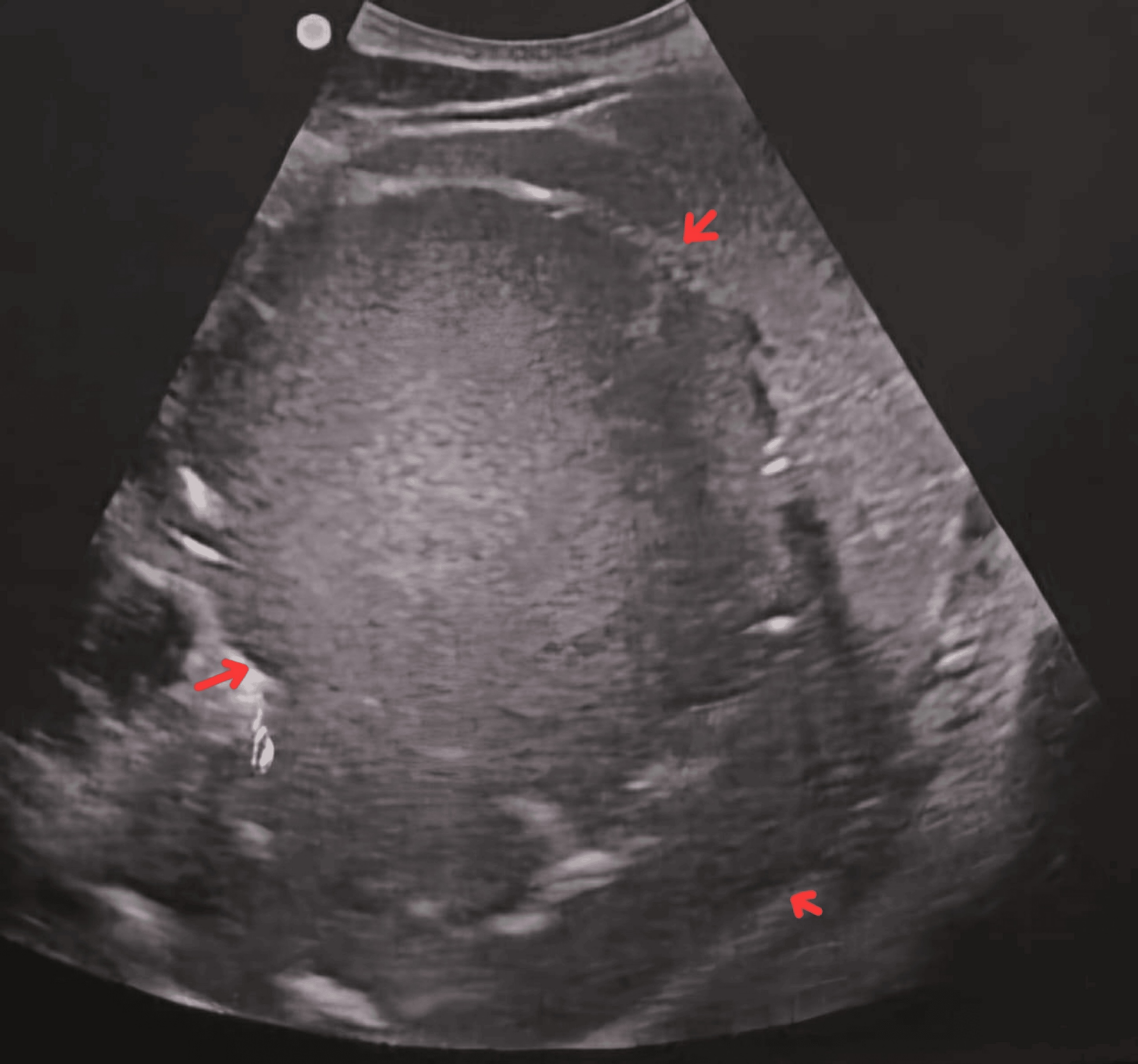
Ultrasonography of the abdomen This image shows a large cystic lesion over the splenic region (red arrows) with laceration over the midpole of the spleen.
